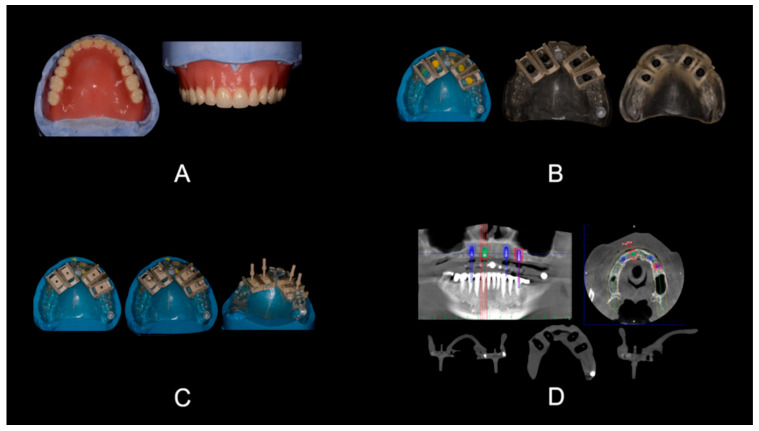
Preoperative planning and fabrication of a prosthesis-based surgical guide system for maxillary implant placement. (A) Original complete denture used as the basis for guide design; (B) duplicate denture with embedded polyether-ether-ketone (PEEK) rails to enable repositioning of the guide sleeve; (C) duplicate denture with directional indicators and adjustable guide sleeve showing angulation options (0°, 12°, and 24°); (D) 3D planning based on cone-beam computed tomography (CBCT) with radiopaque fiduciary markers, enabling prosthetically driven implant trajectory selection.
